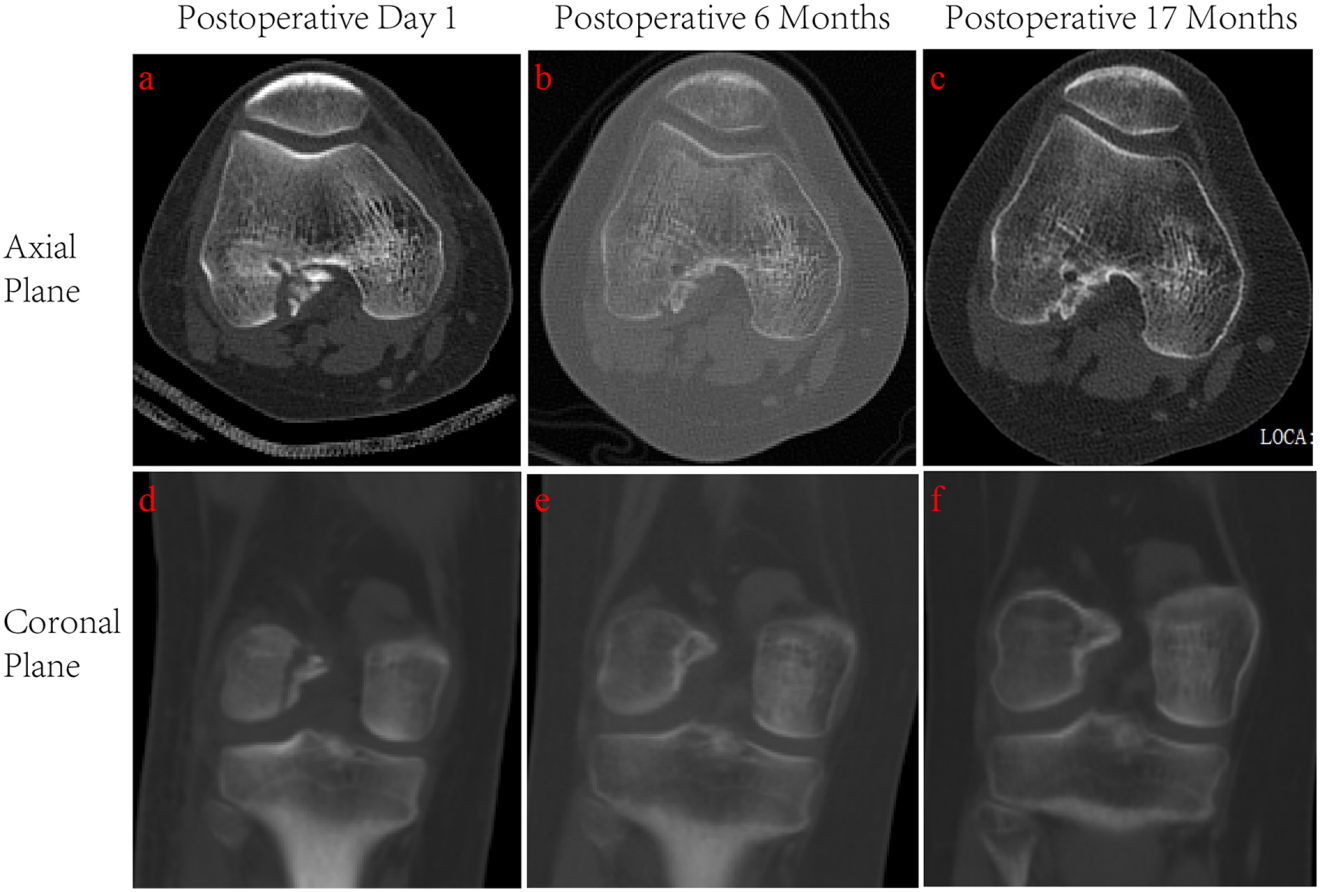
Postoperative CT imaging of the ACL femoral avulsion fracture at three follow-up intervals. (a) Postoperative Day 1, axial view. (b) 6-month follow-up, axial view. (c) 17-month follow-up, axial view. (d) Postoperative Day 1, coronal view. (e) 6-month follow-up, coronal view. (f) 17-month follow-up, coronal view.
